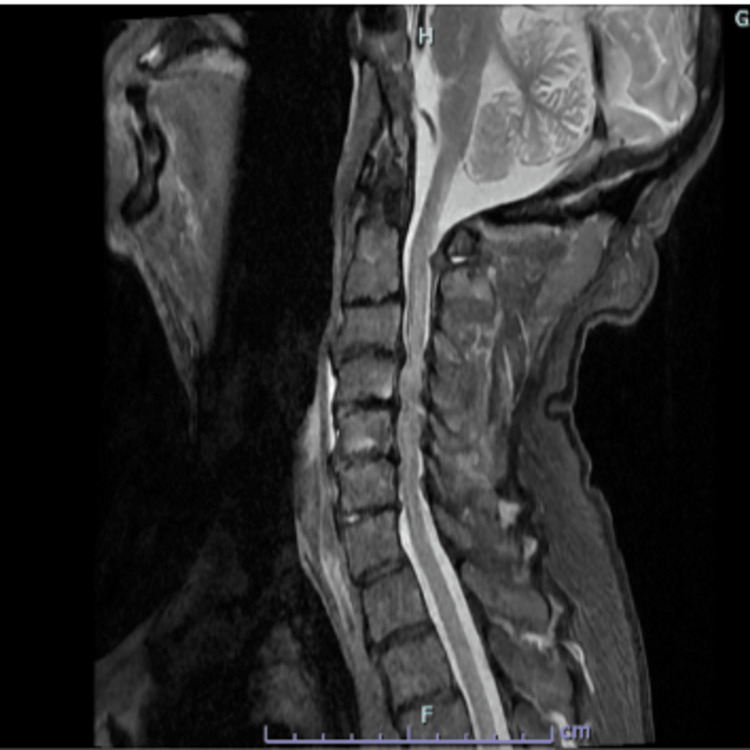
MRI of the cervical spine without contrast demonstrating central canal stenosis at levels C3-C4 and C4-C5.
